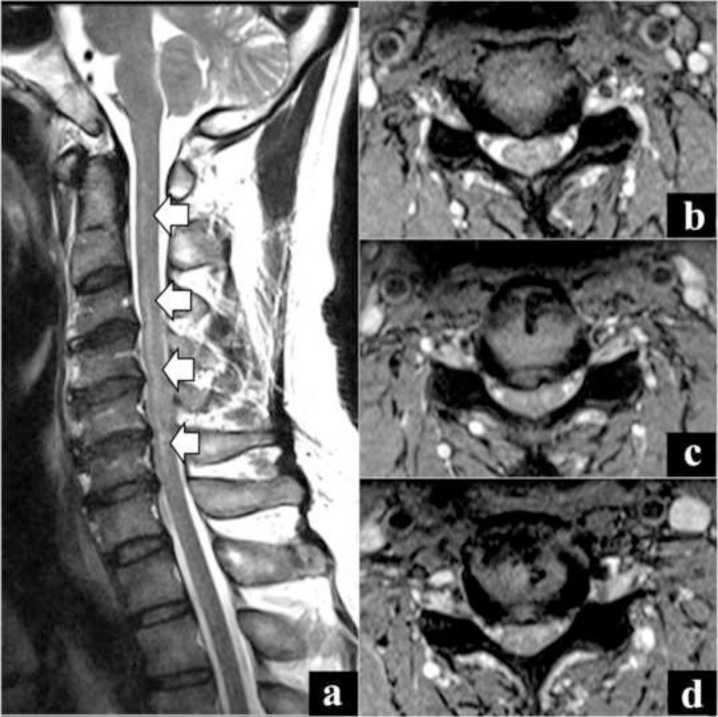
Third MR image demonstrating significant cord signal changes (T2 hyperintensities) from C2 to C7 levels. a) Sagittal cut image, b) Axial cut at C3–C4 level, c) Axial cut at C4–C5 level, d) Axial cut at C5–C6 level.
